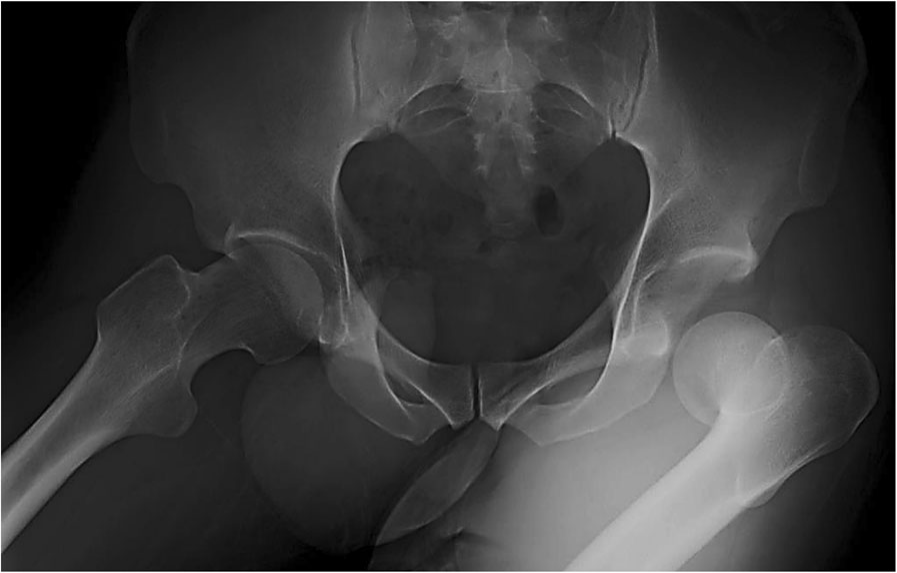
A plain radiograph shows an Epstein–Thompson type 1 posterior dislocation of the left hip with no fracture
